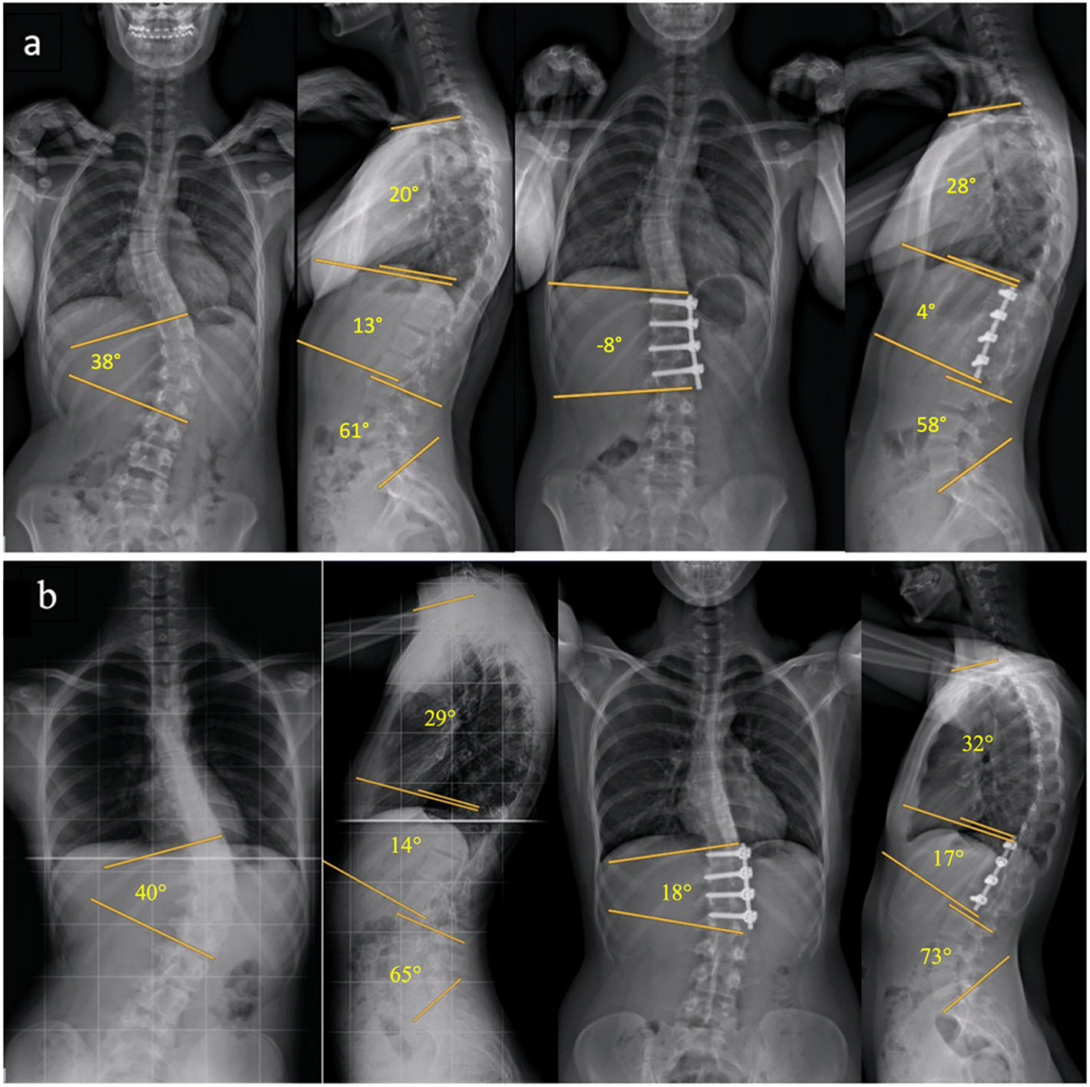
a Standing PA and lateral radiographs with pre- and postoperative Cobb angles in a patient operated with autograft fulcrum effect. b Standing PA and lateral radiographs with pre- and postoperative Cobb angles in a control patient operated without autograft fulcrum effect
